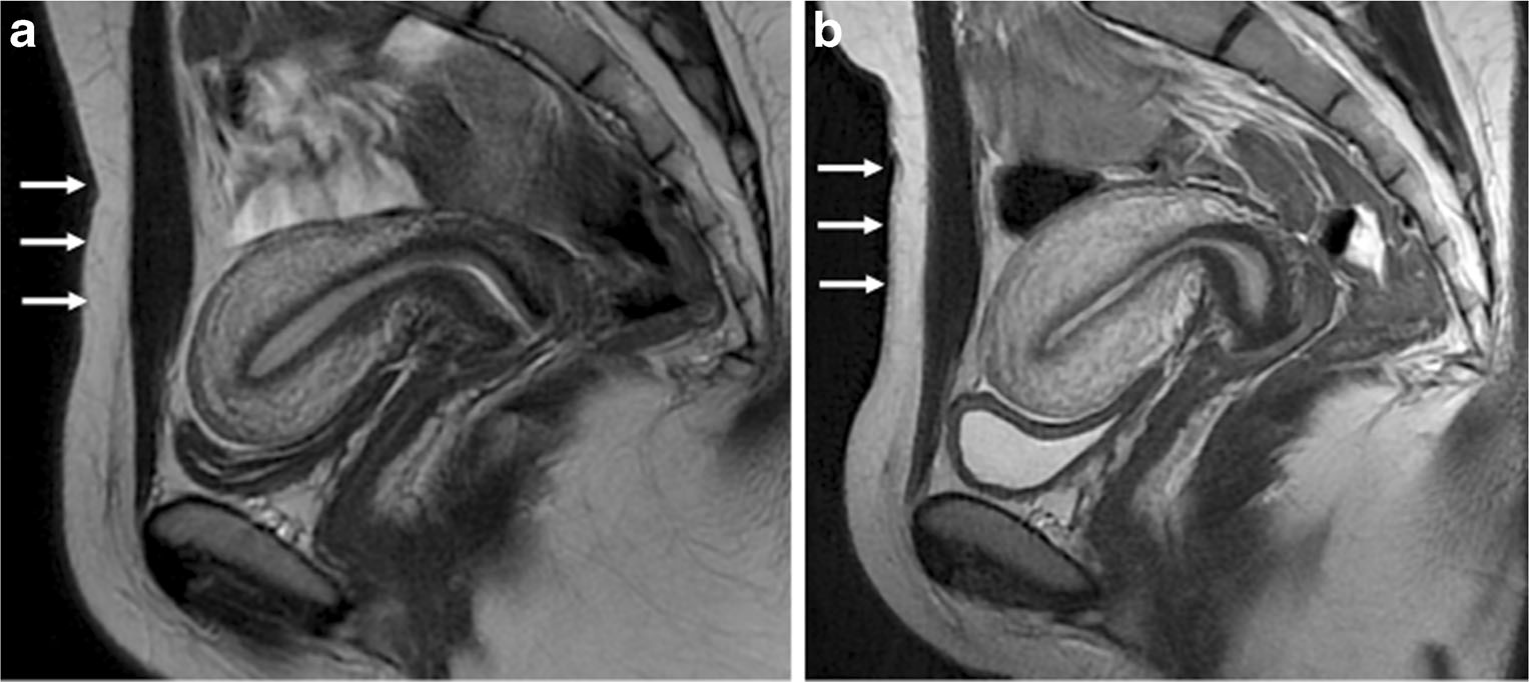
Sagittal 2D T2-weighted MR images in the same patient performed at (a) 1.5 Tesla and (b) 3.0 Tesla provide similar good imaging quality for the evaluation of pelvic anatomy, especially uterine zonal anatomy. Note the quality of abdominal strapping on both 1.5 and 3.0 T examinations (arrows)
